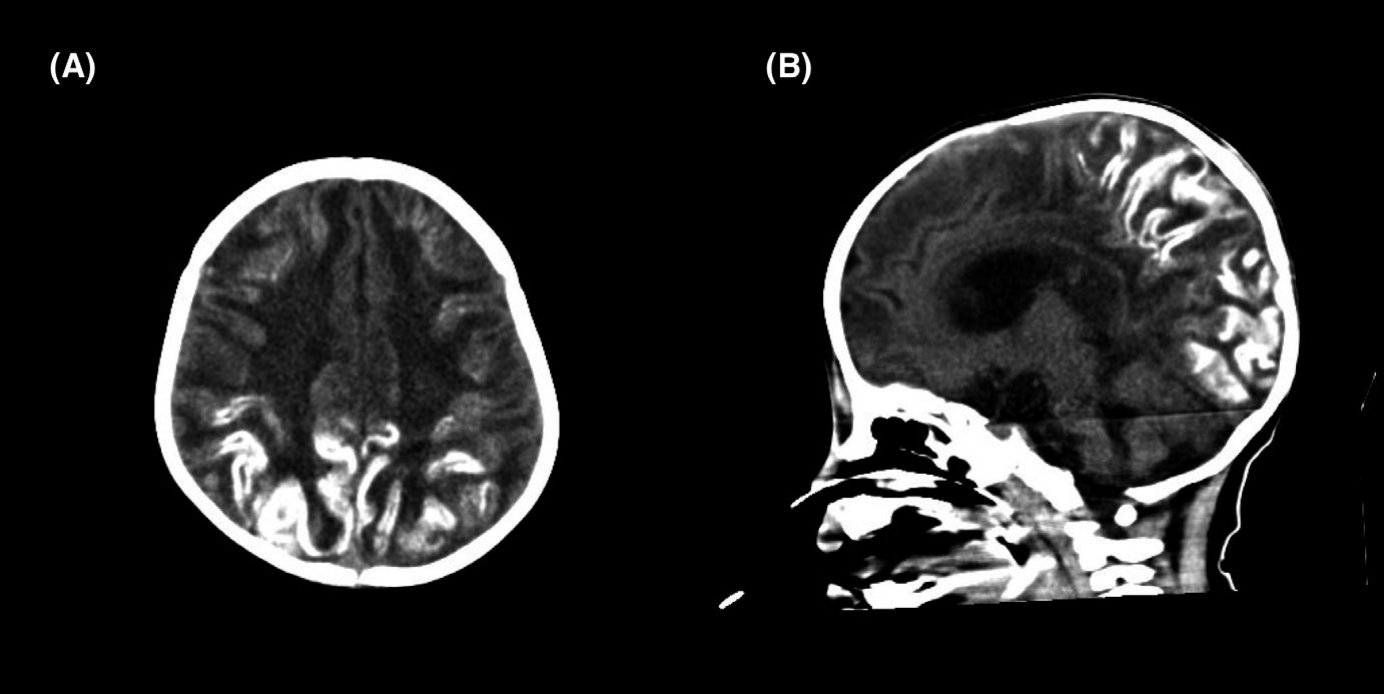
A, Axial computed tomography performed 1 mo after admission showing high‐density lesions in the temporoparietal regions. B, Sagittal computed tomography showing high‐density lesions in the parieto‐occipital regions
